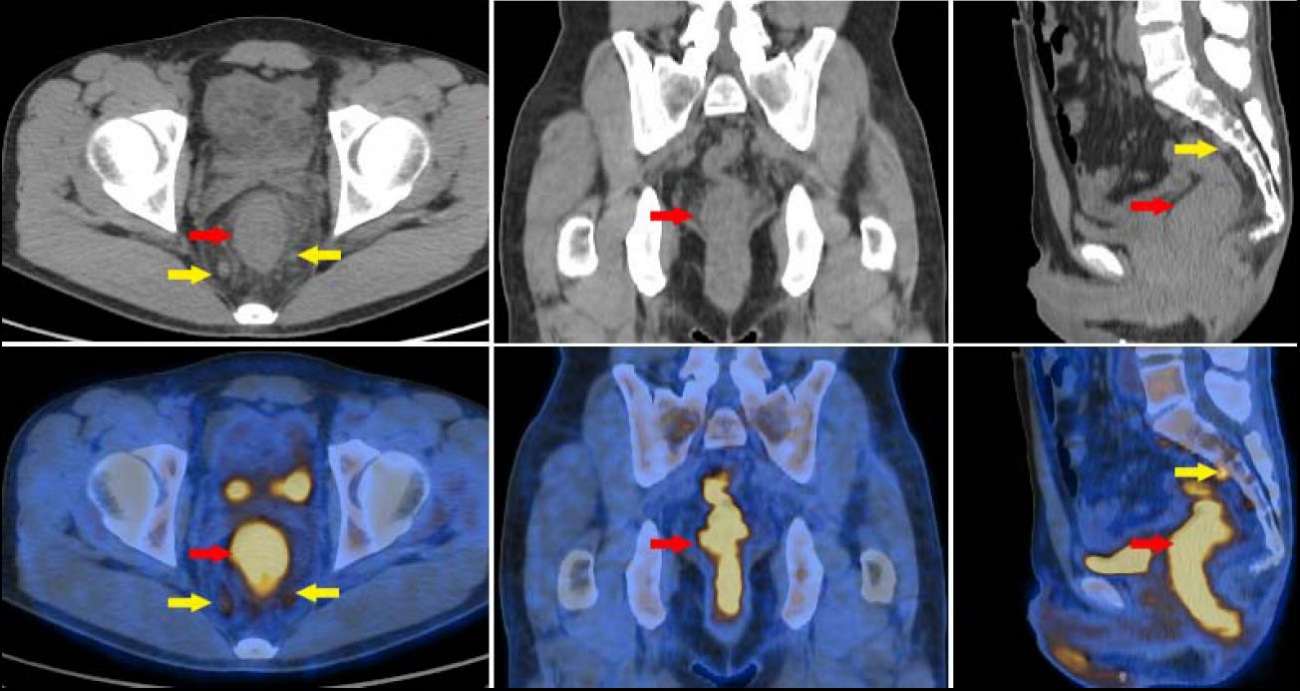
^18^F-FDG PET/CT imaging in patients with CMV colitis. PET/CT imaging displayed diffuse rectum wall thickening and increased glucose metabolism,and the SUVmax was 12.7(red arrow). There were multiple enlarged lymph nodes around the rectum, some of them were enlarged, the short diameter of the larger one was 0.8cm, glucose metabolism was increased, and the SUVmax was 4.6(yellow arrow).
